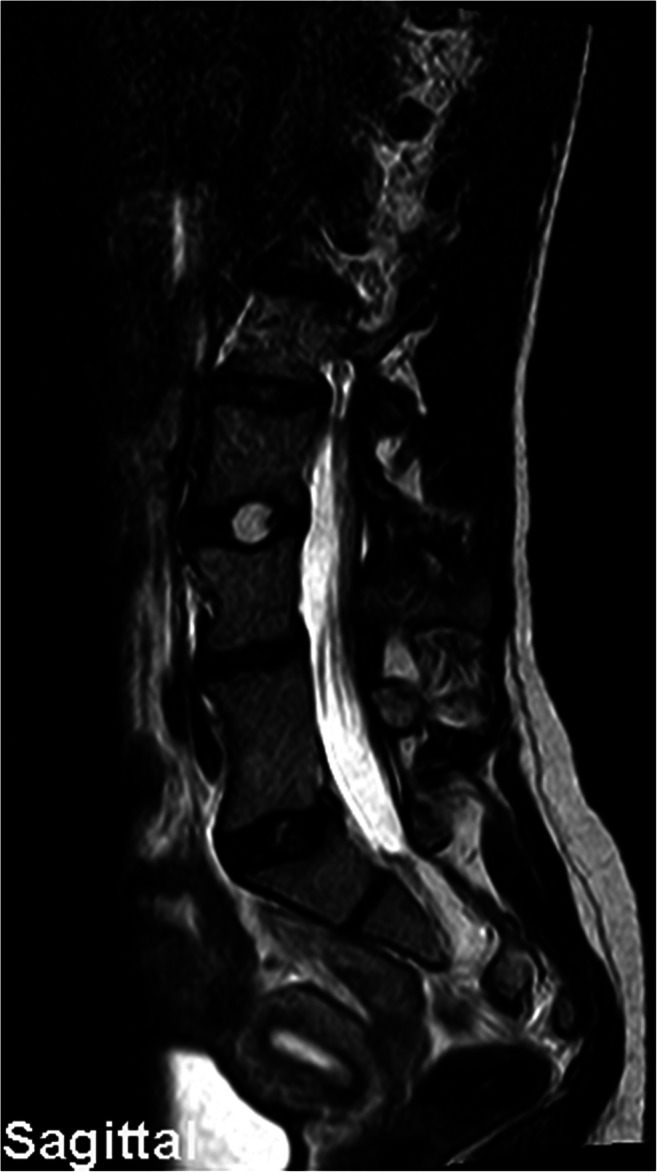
Preoperative imaging. MRI sagittal plane
